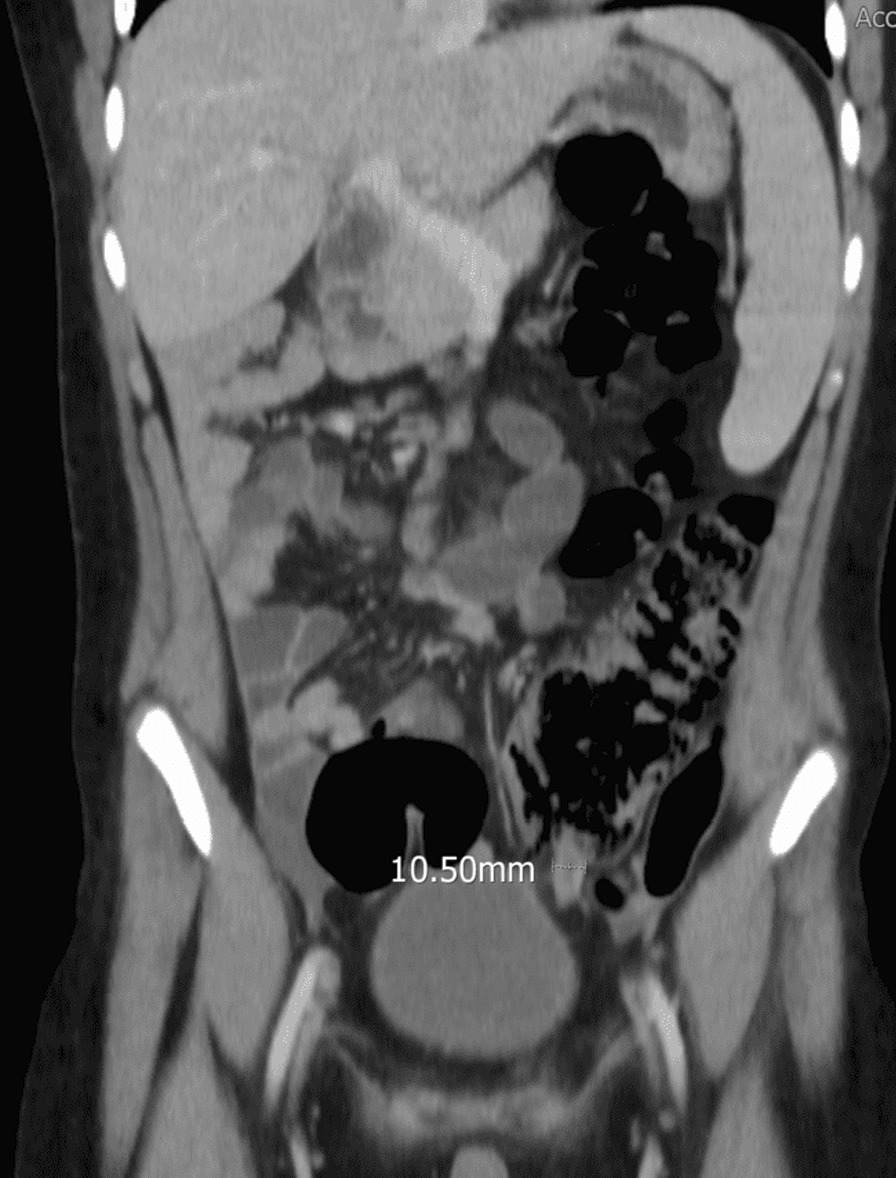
CT of the abdomen showing enlarged appendix (10.50 mm) located to the left of the urinary bladder
